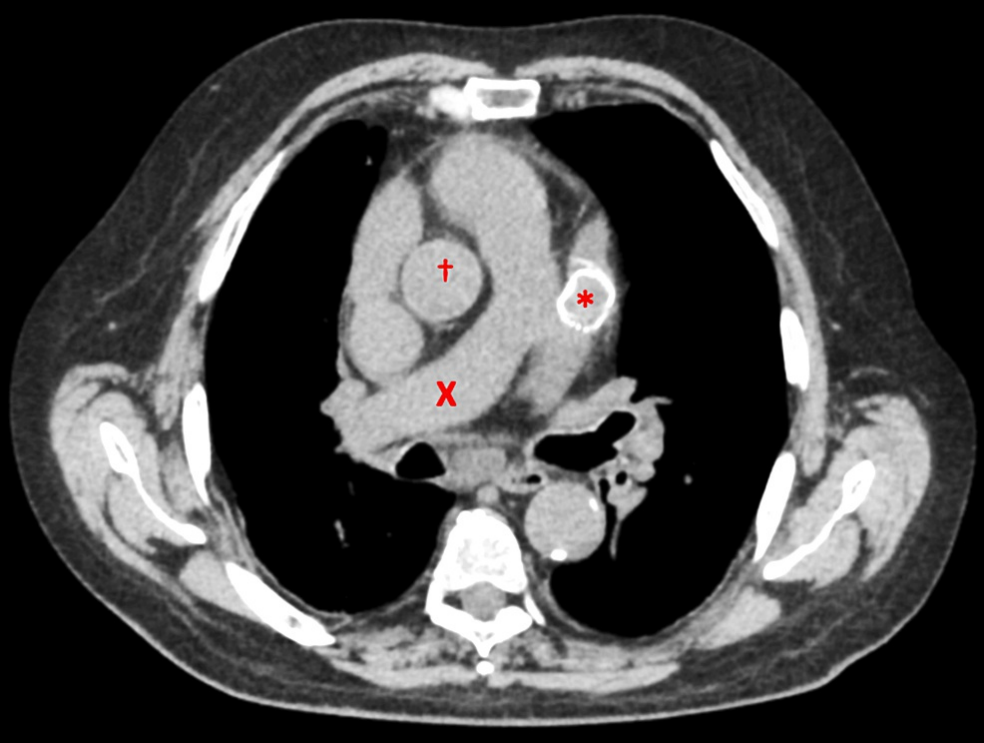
Chest CT showing the Watchman FLX positioned in the left atrial appendage. * = 27 mm Watchman FLX; X = pulmonary artery; † = aorta
